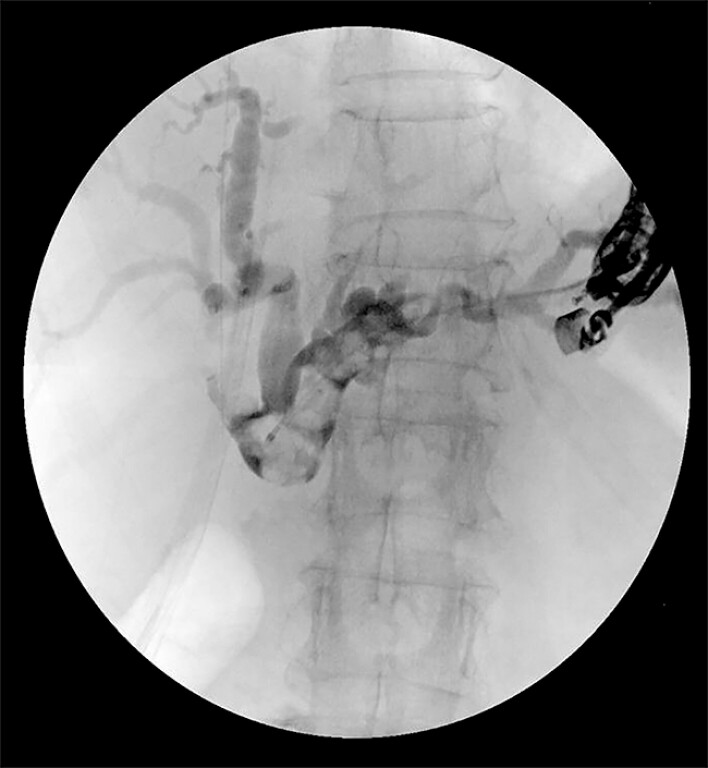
Endoscopic ultrasound-guided hepaticogastrostomy and antegrade cholangiography.
